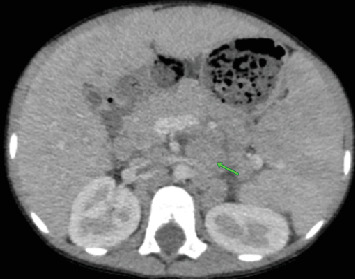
Prechemotherapy axial CT image showing a 1.2 × 2.8 cm homogeneously enhancing retroperitoneal lymph node (arrow).
